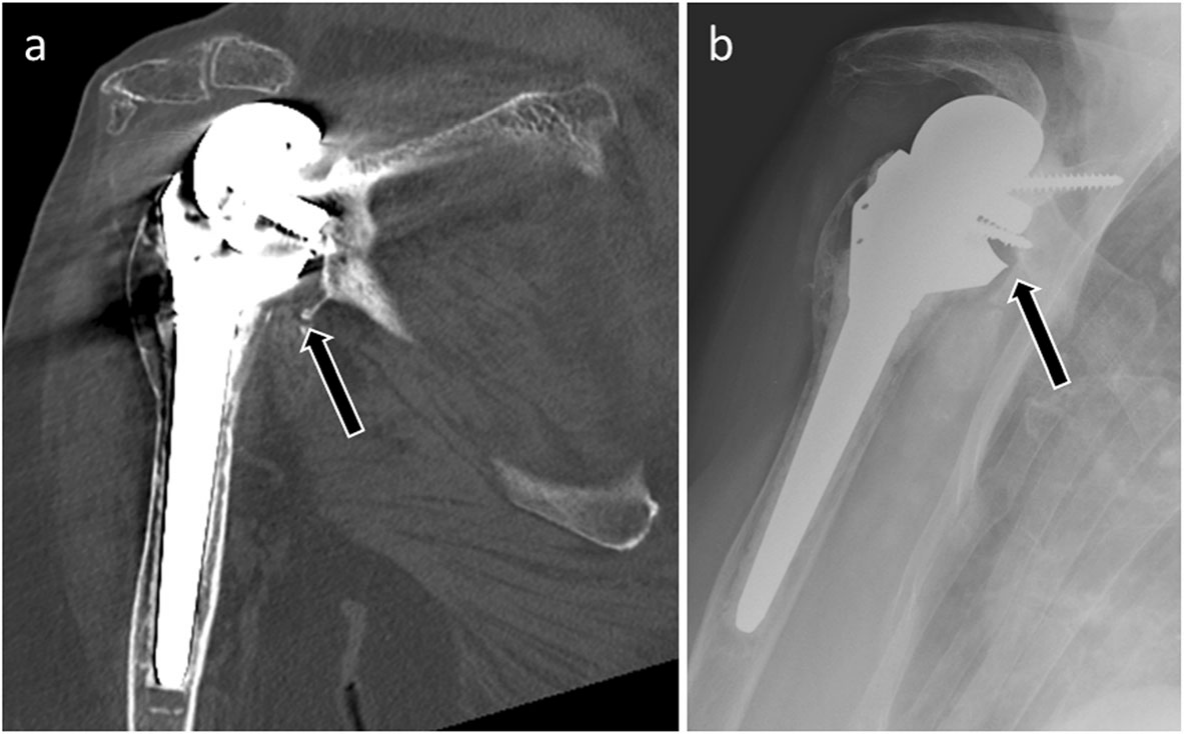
Coronal CT image (a) and anteroposterior radiograph (b) of an 89-year-old patient with painful reverse shoulder arthroplasty show scapular notching manifested by bone resorption of the inferior scapular border (arrows)
